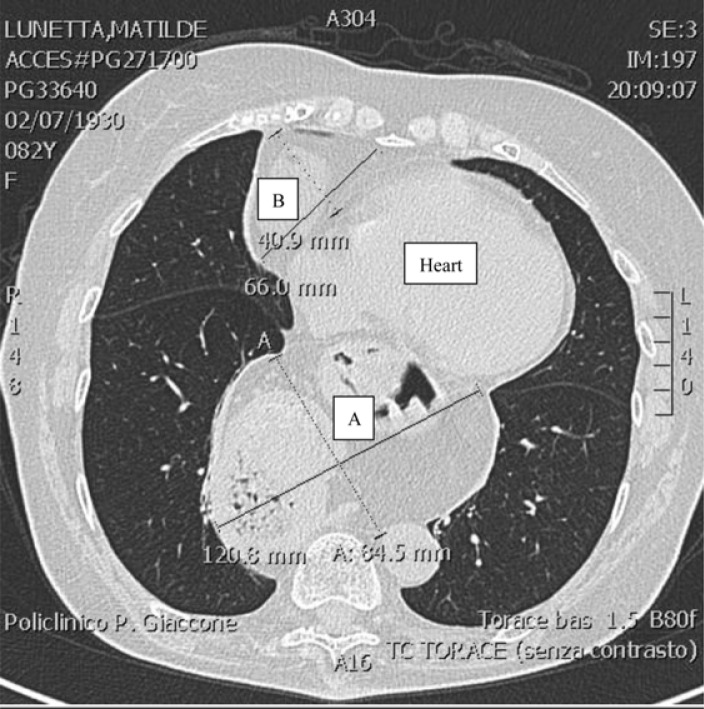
Chest computed tomography demonstrating the complete hiatal hernia compressing right atrium and ventricle with dimensions (A). In the lower retrosternal right side, a diaphragmatic hernia with fluid and adipose content is also present (B).
